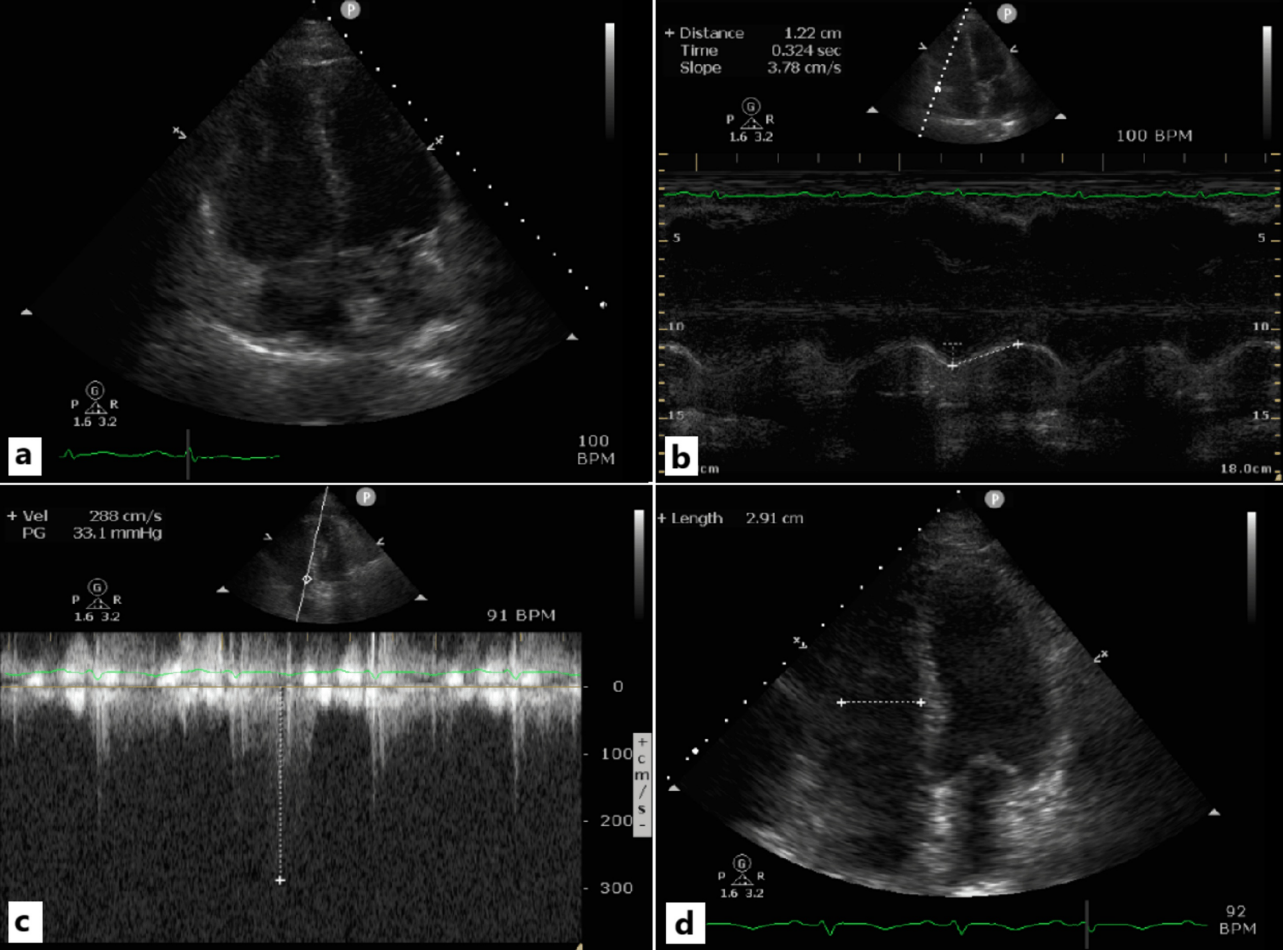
a. Marked enlargement of the RV (apical four-chamber view). Specifically, the ratio of the RV basal diameter to that of the left ventricle is >1. This constitutes a classic echocardiographic marker of RV dysfunction and/or pressure overload in the setting of APE. The measurement was obtained at the time of APE diagnosis. b. Reduced RV contractility based on the TAPSE measurement (apical four-chamber view). The measurement was obtained at the time of APE diagnosis. c. Estimated PASP calculated from the maximal tricuspid regurgitation velocity during the recovery phase (follow-up TTE). PASP is reduced, compared with the estimated value at APE diagnosis, reflecting the effectiveness of therapy in thrombus resolution. d. The estimated mid-cavity diameter of the RV in the apical four-chamber view during the recovery phase is within normal limits. A significant overall reduction in RV dimensions is also evident. RV: Right ventricle/ventricular, APE: Acute pulmonary embolism, PASP: Pulmonary artery systolic pressure, TAPSE: Tricuspid annular plane systolic excursion, TTE: Transthoracic echocardiogram
